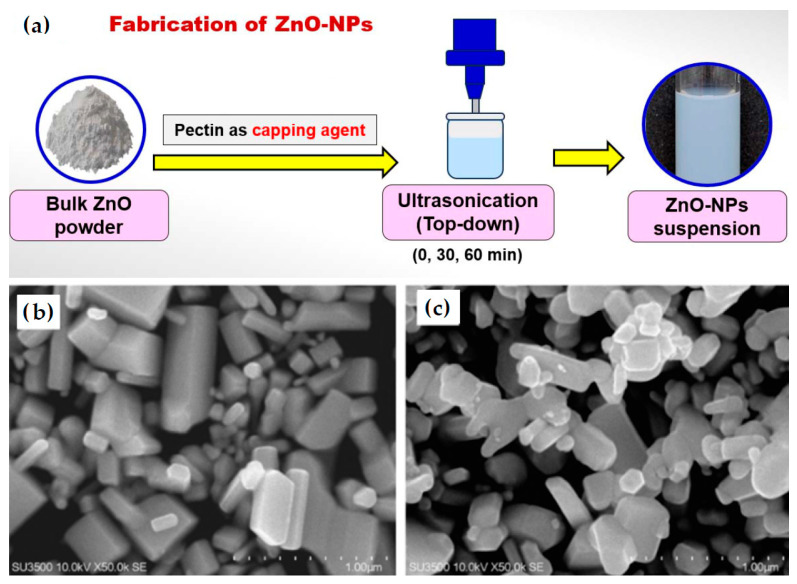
Pectin-capped ZnO NPs obtained through ultrasonication in double-distilled water: (a) a scheme of the natural gum-mediated fabrication method and SEM images of ZnO-NPs after ultrasonication for (b) 30 m and (c) 60 m (reproduced from [90]).
